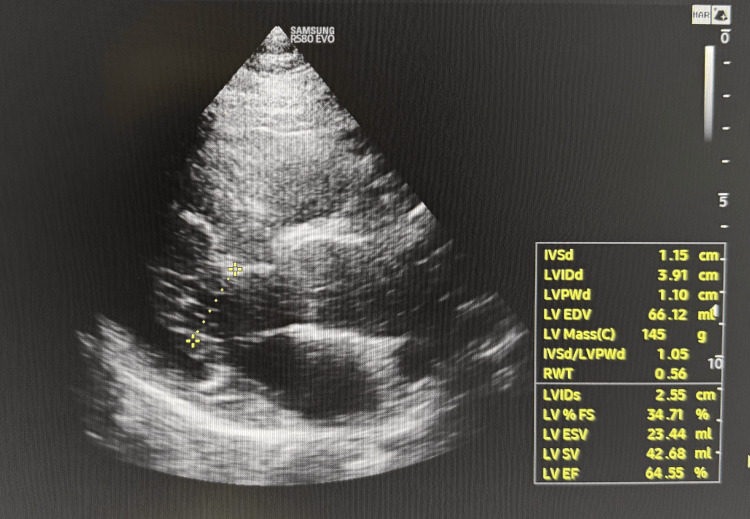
Transthoracic echocardiogram showing mild concentric left ventricular hypertrophy.
